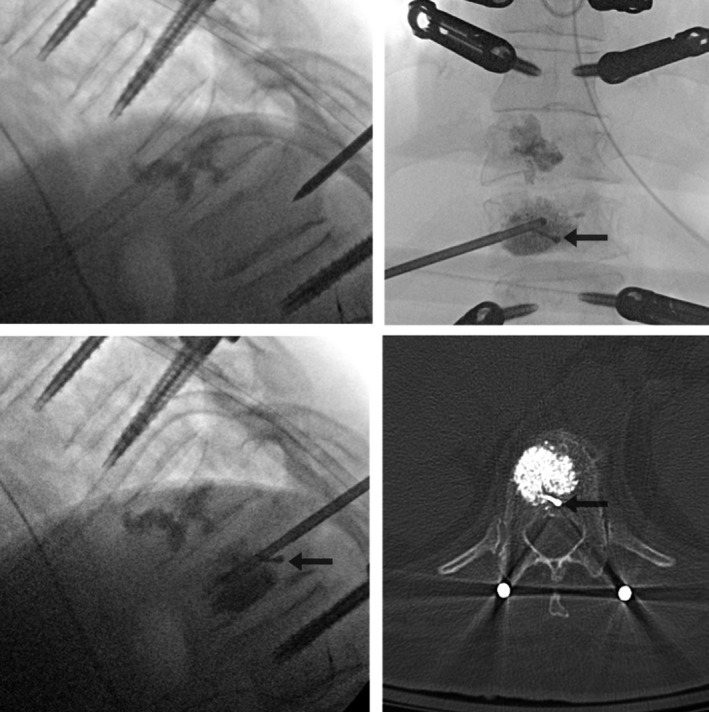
Intraoperative fluoroscopy after minimally invasive placement of pedicle screws T8‐L1. Left top: the Jamshidi needle is being advanced into the T11 vertebral body. Right top and left bottom: AP and lateral fluoroscopy, respectively, demonstrating the broken distal tip of the kyphoplasty curette. Bottom right: Axial slice of CT‐scan at the level of T11 vertebral body showing the broken tip of the kyphoplasty curette
